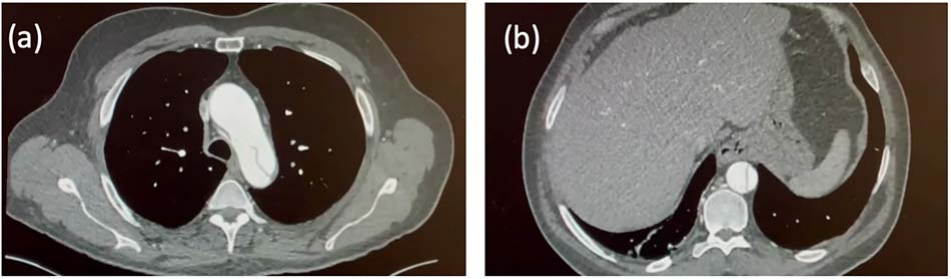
Initial CT aortogram. Aortic dissection originating from the origin of left subclavian artery with the ascending aorta measuring 45 mm (a). The dissection extends to involve the descending aorta and abdominal aorta (b).
